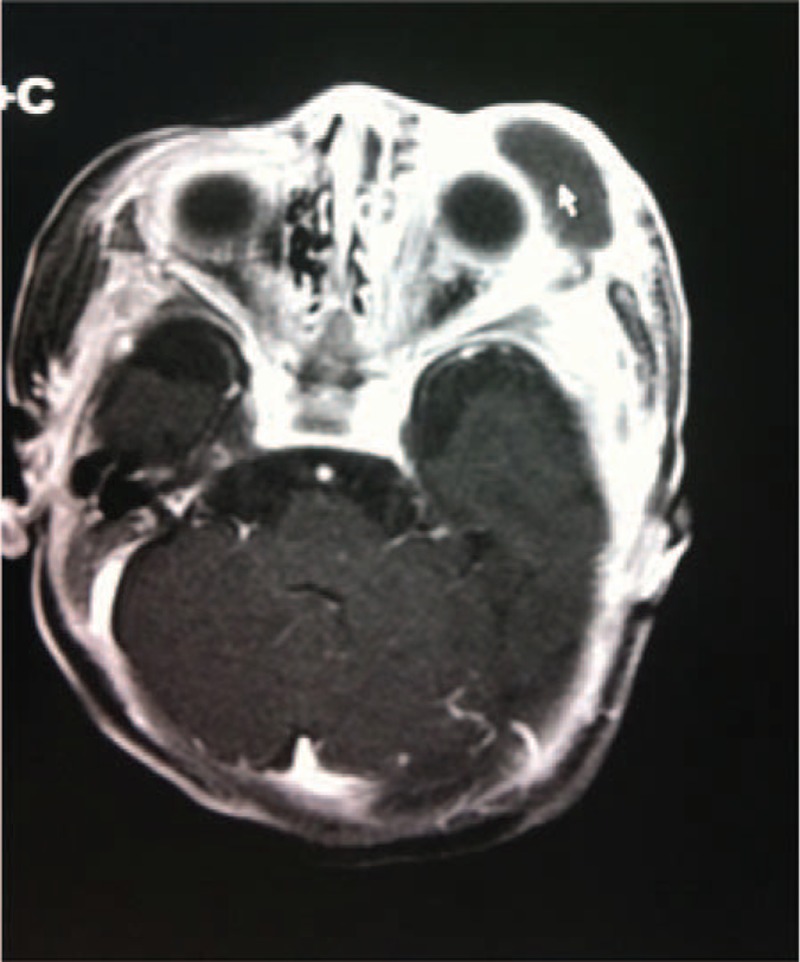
Enhanced MRI showed swelling soft tissue around left orbit, and abscess (indicated by arrows). The signal on bone marrow of external wall of left orbit was intensified. MRI, magnetic resonance imaging.
